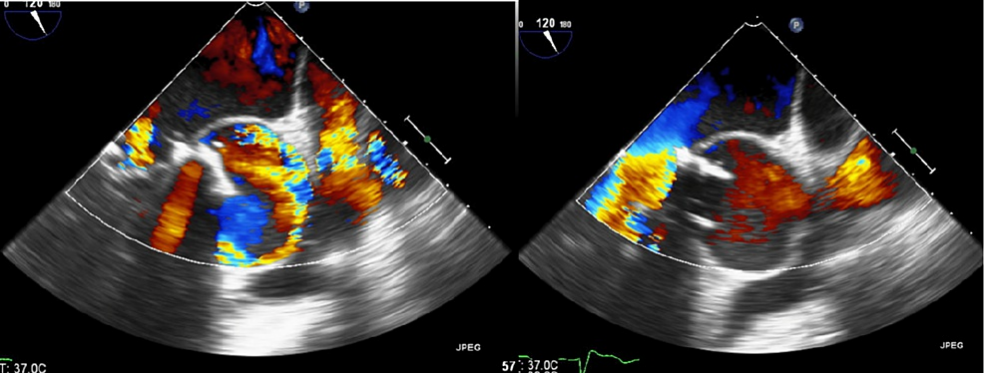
Transesophageal echocardiogram. The images are showing regurgitation through the prosthetic aortic valve and aortic root pseudoaneurysm.
